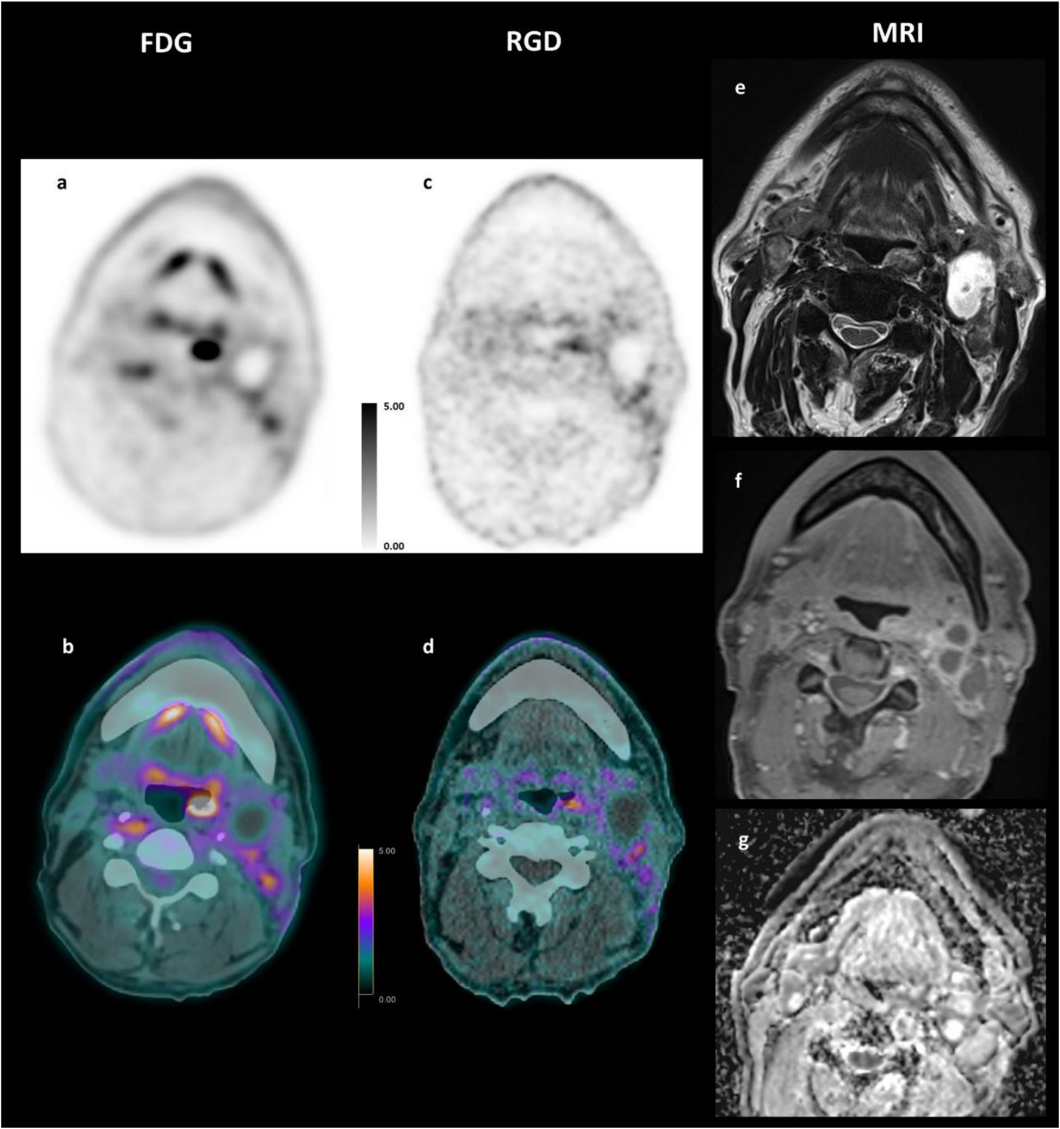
Comparative ^18^F-FDG (a, b), ^68^Ga-NODAGA-RGD PET/CT (c, d), and MRI (e T2w axial, f T1w post Gadolinium, g ADC map of diffusion), of patient #9 (59-year man with moderate differentiated left tonsil squamous cell carcinoma). The ^18^F-FDG and ^68^Ga-NODAGA-RGD PET images showed different signal-to-noise ratios and a slightly different distribution of activity within the tumor bed. The left cervical lymph node showed a photopenic center with absence of tracer uptake for both tracers corresponding to necrosis on MRI
